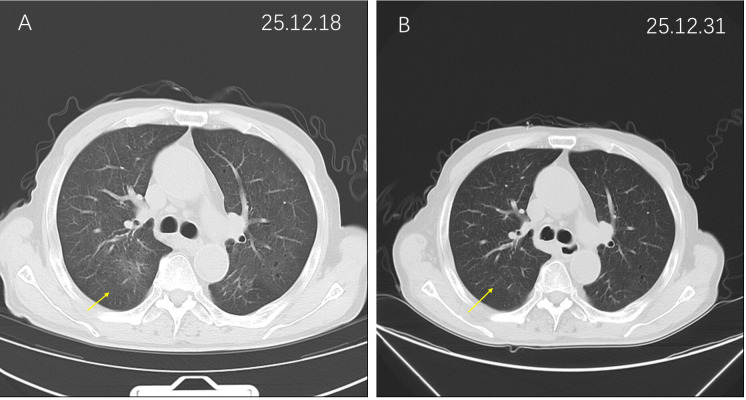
Chest computed tomography (CT) findings of immune-related pneumonitis before and after corticosteroid therapy. Chest CT demonstrated radiographic changes consistent with immune-related pneumonitis and the subsequent treatment response. (A) Chest CT at symptom onset during corticosteroid tapering indicated bilateral pulmonary infiltration with patchy ground-glass opacities and interstitial changes, predominantly in the lower lung fields, which was consistent with immune-related pneumonitis. (B) Follow-up chest CT performed after corticosteroid re-escalation demonstrated a marked resolution of the previously recorded pulmonary infiltration, with only minimal residual fibrotic changes, indicating a favorable radiographic response to immunosuppressive therapy.
